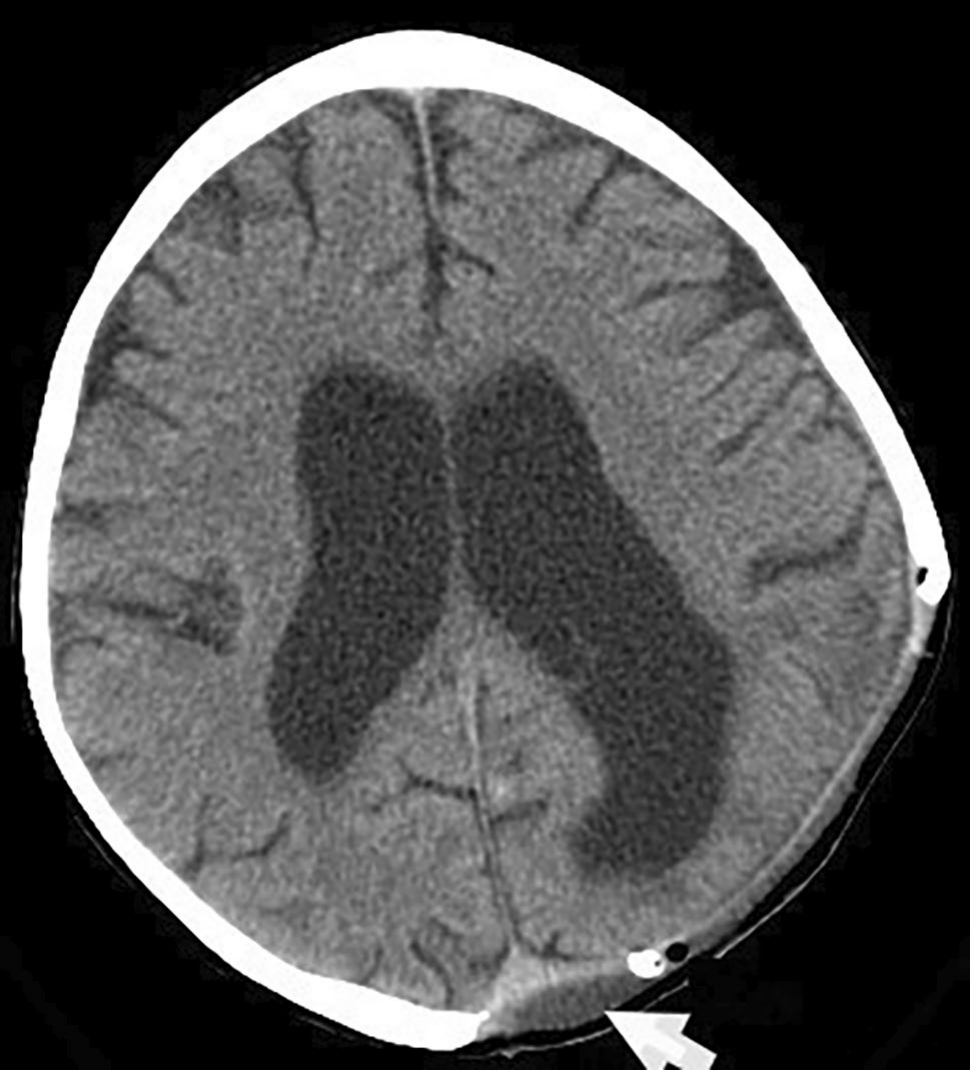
Axial postoperative non enhanced CT scan in parenchymal window performed 2 days postoperatively showing a subcutaneous collection next to the surgical site (white arrow) with an air bubble. Note the disappearance of the extra parenchymal cystic mass and its membranes
